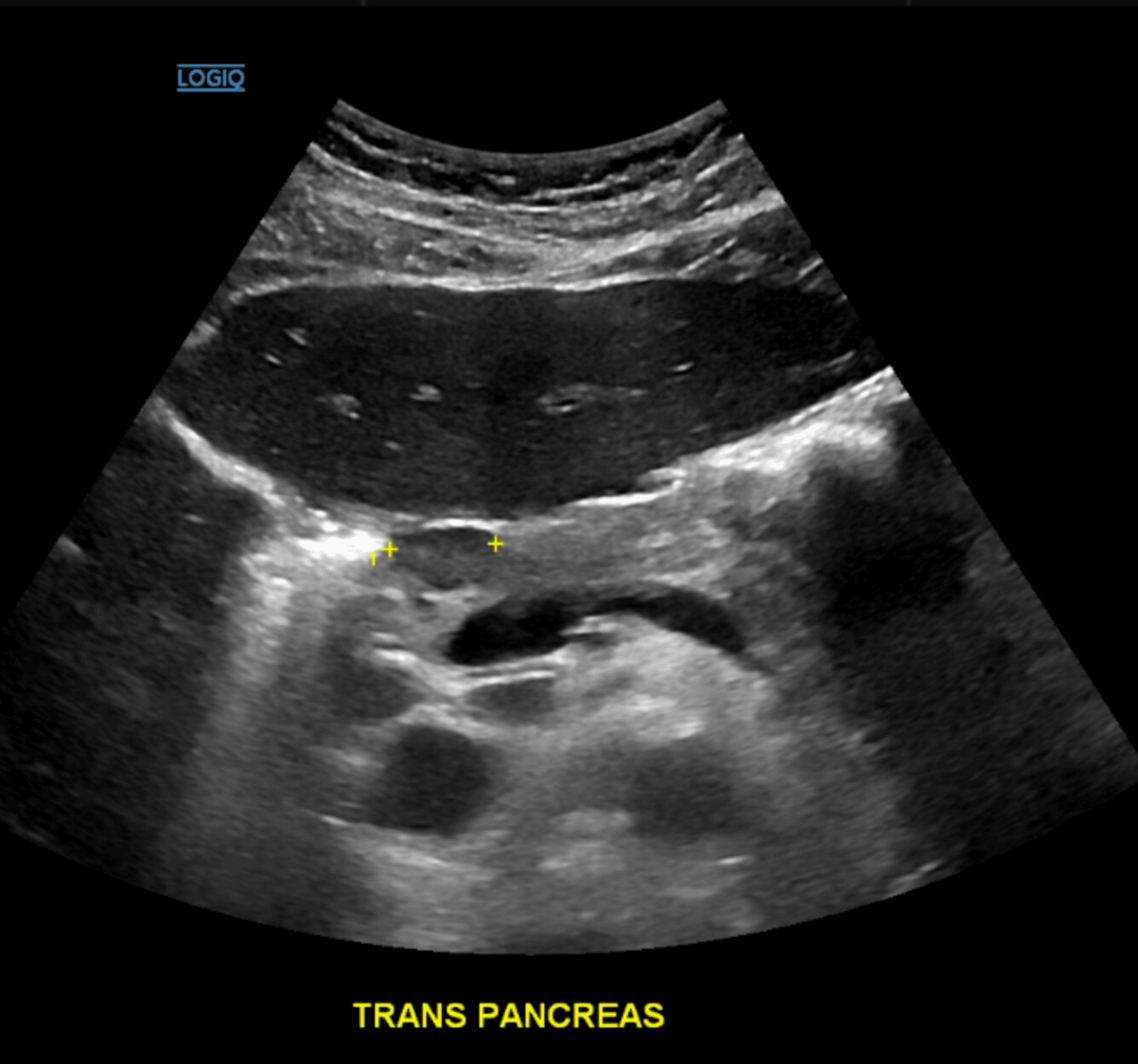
Abdominal ultrasound demonstrating a nodular density at the pancreatic head, shown by yellow markings on the image
